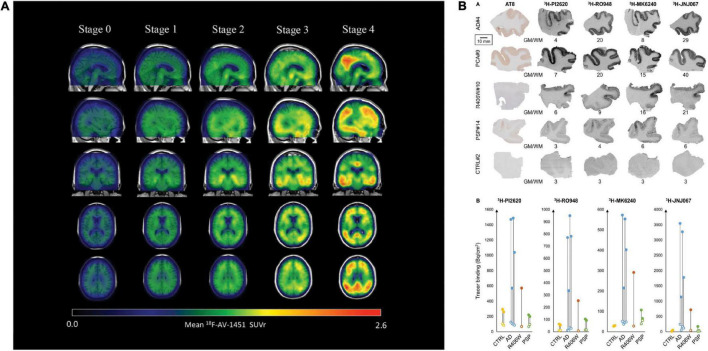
Disease staging with the tau PET tracer [^18^F]AV1451 and autoradiography with [^3^H]PI-2620, [^3^H]MK6240, [^3^H]RO948, and [^3^H]JNJ067. (A) Representation of tau PET images labeled with flortaucipir ([^18^F]AV1451) for staging tau pathology. An increase in SUVR is visible in the cortex and subcortex from stage 0 to 4. Stage 0 represents tau levels of healthy controls. In stage 1, tau levels are elevated in medial temporal areas. [^18^F]AV1451 accumulates in extramedial temporal regions in stage 2, followed by higher SUVRs in the inferior and lateral temporal lobes in stage 3. Significantly increased uptake finally occurred in the neocortex in stage 4. Reproduced from Chen et al. (2021) with permission from Springer Nature. (B) Characteristics of the binding properties of the tau tracers [^3^H]PI-2620, [^3^H]MK6240, [^3^H]RO948, and [^3^H]JNJ067 in the medial temporal lobe of patients with AD, primary tauopathies and healthy controls. (*R406W* = FTD), here a *MAPT R406W* missense mutation leads to the formation of NTFs in the case of FTD with parkinsonism linked to chromosome 17 (FTDP-17), PCA, posterior cortical atrophy; PSP, progressive supranuclear palsy. Reproduced from Yap et al. (2021) with permission from Oxford press.
